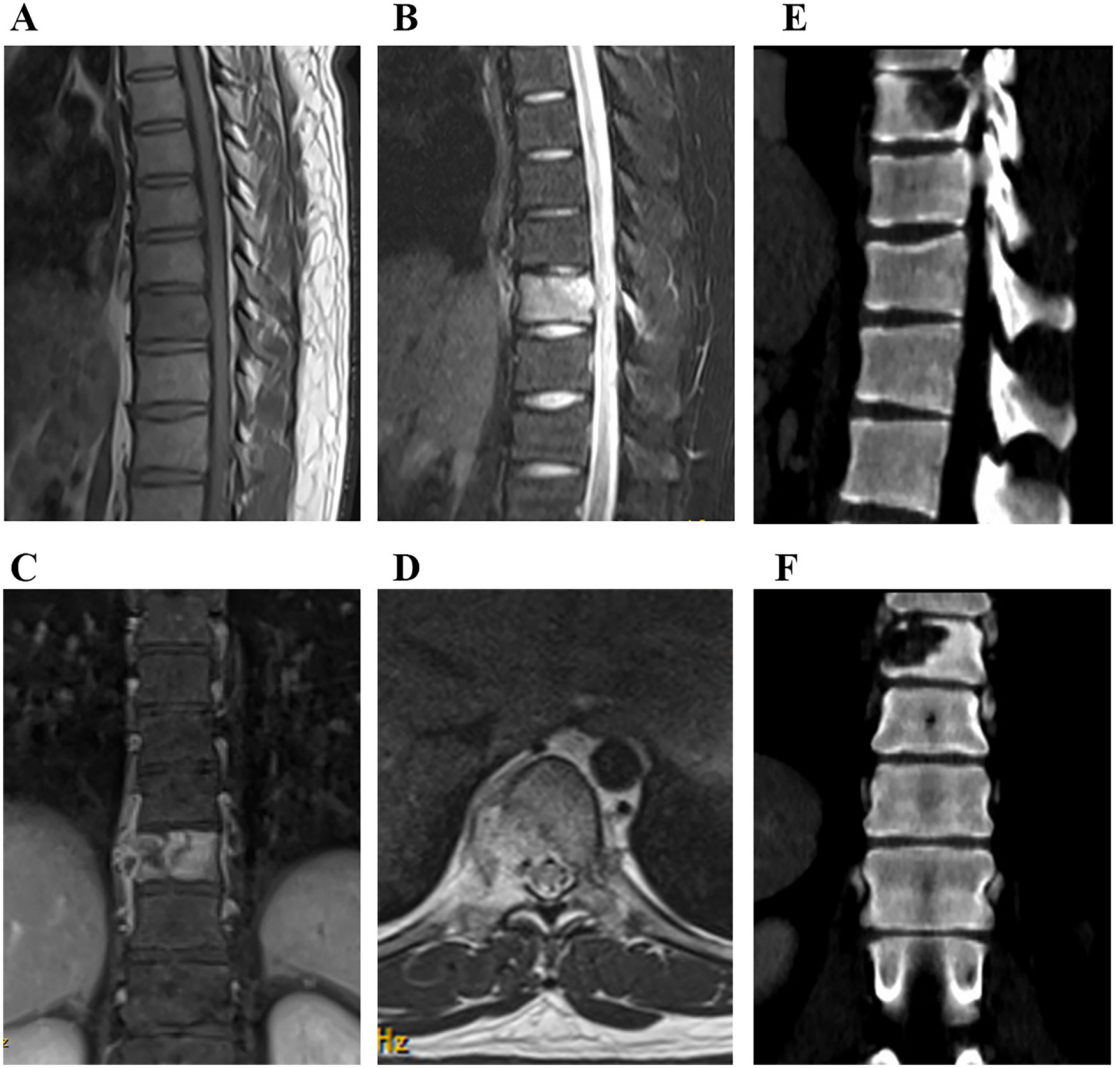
Preoperative MRI (A–D) and CT (E,F) examinations. An occupation lesion, hypointense on T1-weighted images and hyperintense on T2-weighted images, was detected in the ninth thoracic vertebrae.
